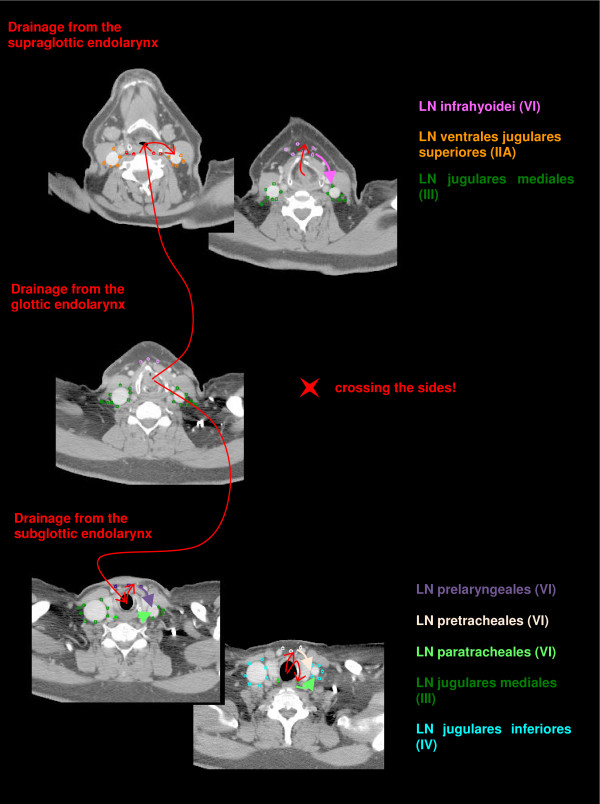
Lymph drainage from the endolarynx contoured in transversal CT slices (red arrows) to the LN infrahyoidei (pink), LN prelaryngeales (violet), LN pretracheales (light pink) and LN paratracheales (light green).
